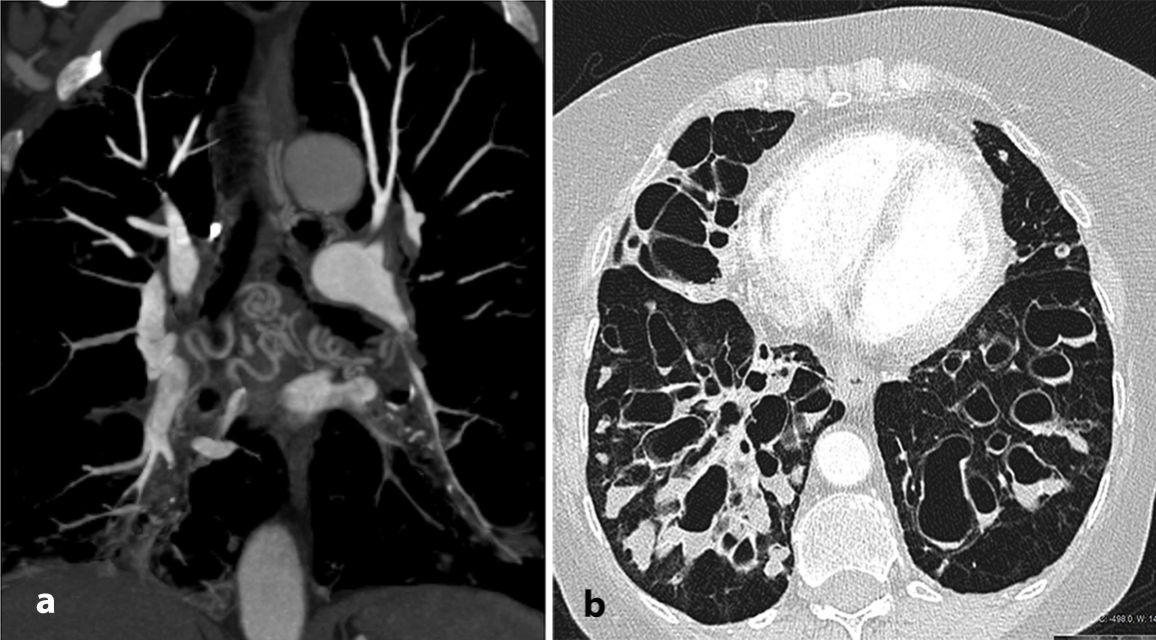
(a) Coronal maximum intensity projection reformat of the mediastinum from the intitial CT pulmonary angiogram shows multiple collateral bronchial arteries supplying the diseased lung. (b) Axial slice of the same CT scan with lung windowing shows severe cystic bronchiectasis, bronchial wall thickening and bronchocoeles/intrabronchial fluid levels.
